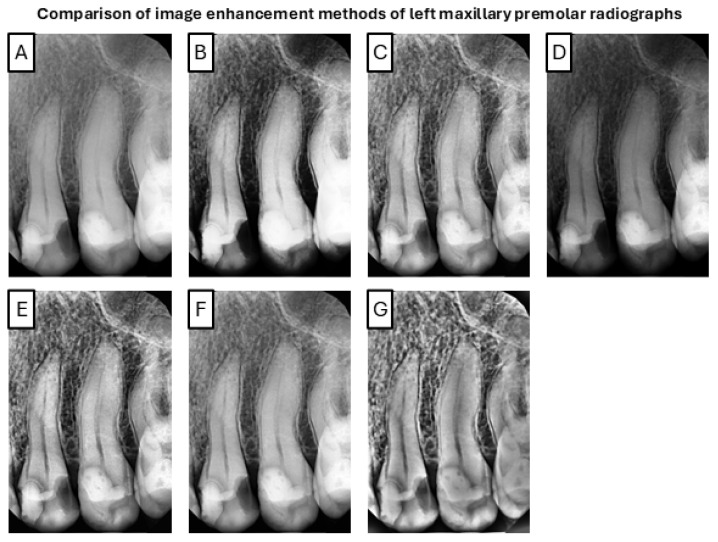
Representative example of premolar radiographs processed using the evaluated enhancement methods. Enhanced images demonstrate varying levels of contrast improvement and anatomical detail visibility. (A) Original, (B) HE, (C) CLAHE, (D) KFCDO, (E) G-CLAHE, (F) CLAHE-POA, (G) TV-CLAHE.
